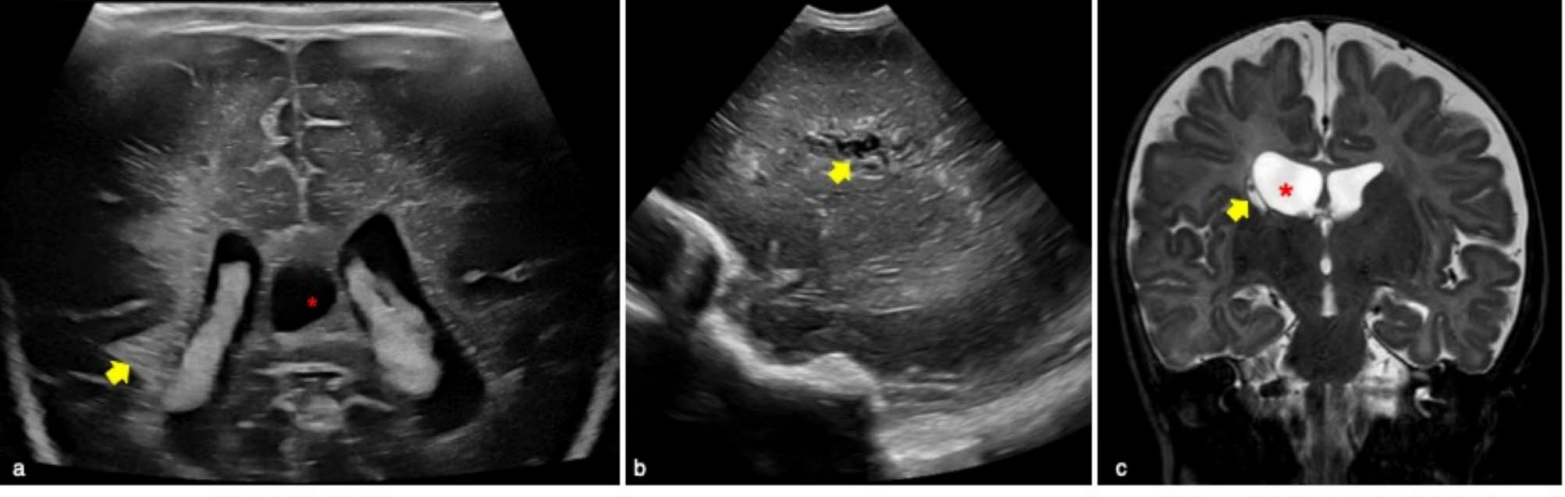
Early and late phases evolutions of periventricular leukomalacia of premature infants. a Third-day of life cerebral ultrasound of premature male infant born at 31 weeks and 5 days gestation showed right periventricular white matter hyperechoic areas (yellow arrow), particularly in the right occipital horn. These findings are typical of first 48-h periventricular leukomalacia. Cavum Vergae as anatomical variant is also showed (red asterisk in a). Follow-up radiological imaging of premature female infant born at 33 weeks gestation and birth weight of 1300 g performed three months after first ultrasound examination (not showed). Sagittal ultrasound plane well demonstrated cystic changes of the periventricular white matter (yellow arrow in b). Cystic areas were confirmed on coronal MR T2 weighted, also well demonstrating right ventricular dilatation (yellow arrow and red asterisk in c, respectively). These were typical findings of late-phase evolution of periventricular leukomalacia
